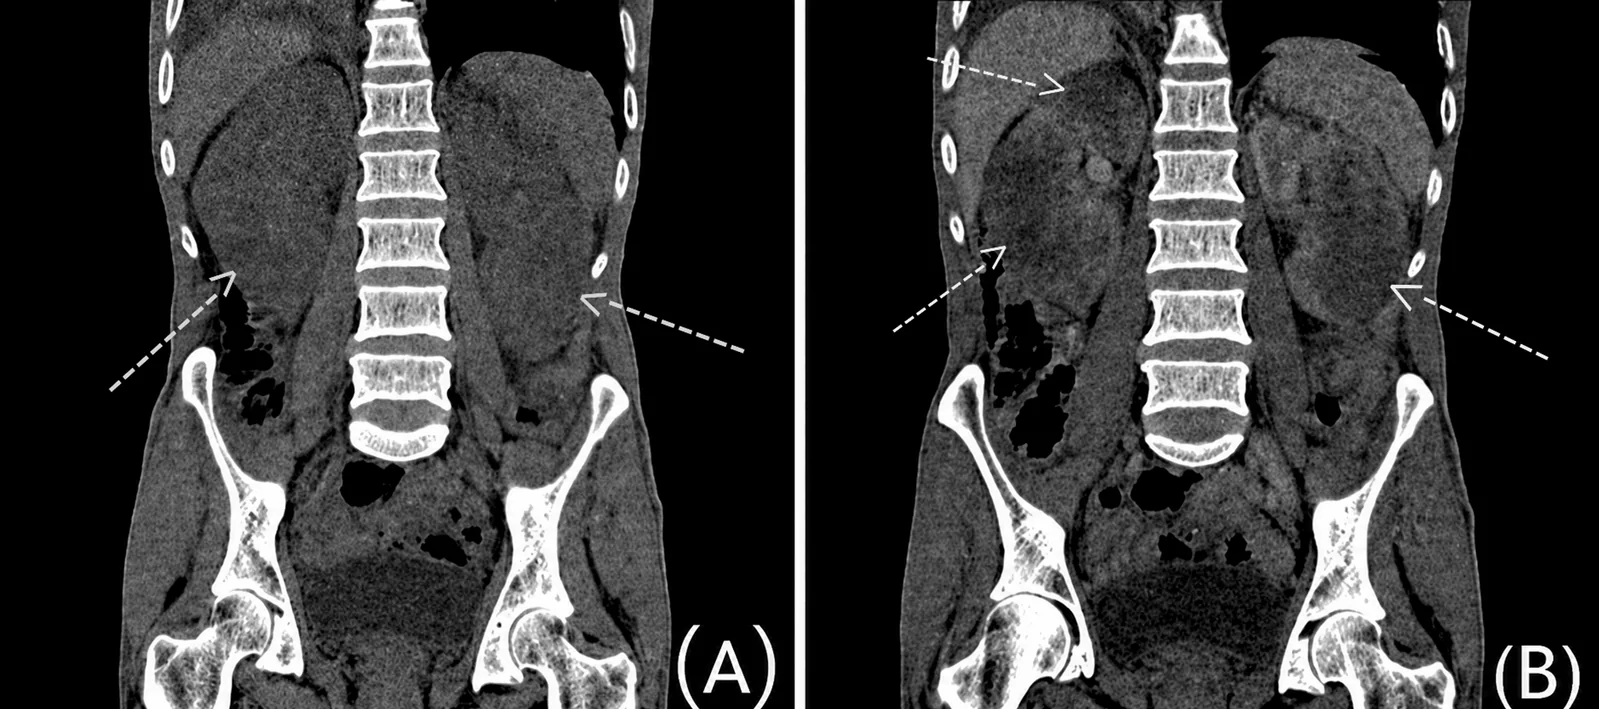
Contrast-enhanced abdominal computed tomography showing bilaterally enlarged kidneys with multiple well-defined hypodense lesions: (A) unenhanced scan and (B) contrast-enhanced scan
